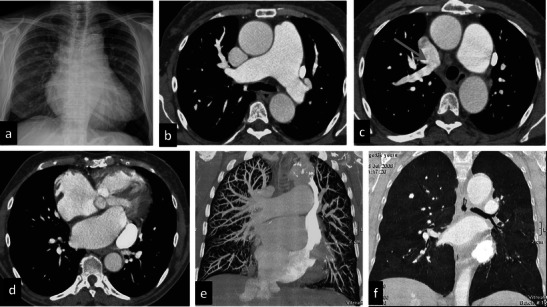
Azygos agenesis. A 64-year-old female with a prior mitral valve cardiac substitution. She underwent the PE protocol because of severe dyspnoea and hypoxaemia. Chest X-ray (a) shows increased pulmonary vasculature and mild dilatation of the upper mediastinum particularly on the left side and enlargement of the mediastinal pedicle. Contrast-enhanced CT shows severe enlargement of the pulmonary artery (b) and anomalous venous return with a right upper lobe pulmonary vein draining into the superior vena cava (c). On the left side a persistent left superior vena cava is seen draining into the coronary sinus (d), which is enlarged, too. Marked dilatation of the right atrium and ventricle can be seen. Azygos and hemiazygos veins are absent, suggesting agenesis. Coronal MIP and MinIP reconstructions (e–f) show an increase of the pulmonary vasculature (e) and moderate mosaic perfusion (f) related to severe pulmonary hypertension
